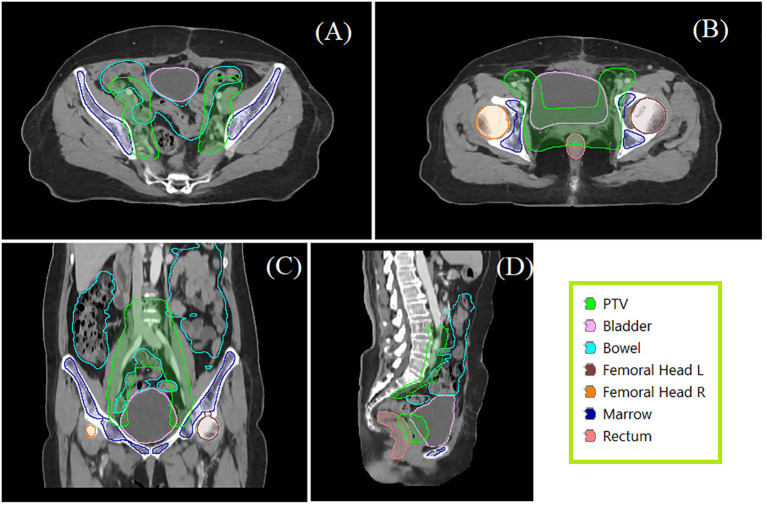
Planning CT images of a representative patient with target and organ-at-risk delineation. The planning target volume (PTV) and relevant organs at risk, including the bladder, bowel, rectum, bone marrow, and bilateral femoral heads, are shown in axial (A, B), coronal (C), and sagittal (D) views. A color-coded legend is provided for clarity.
